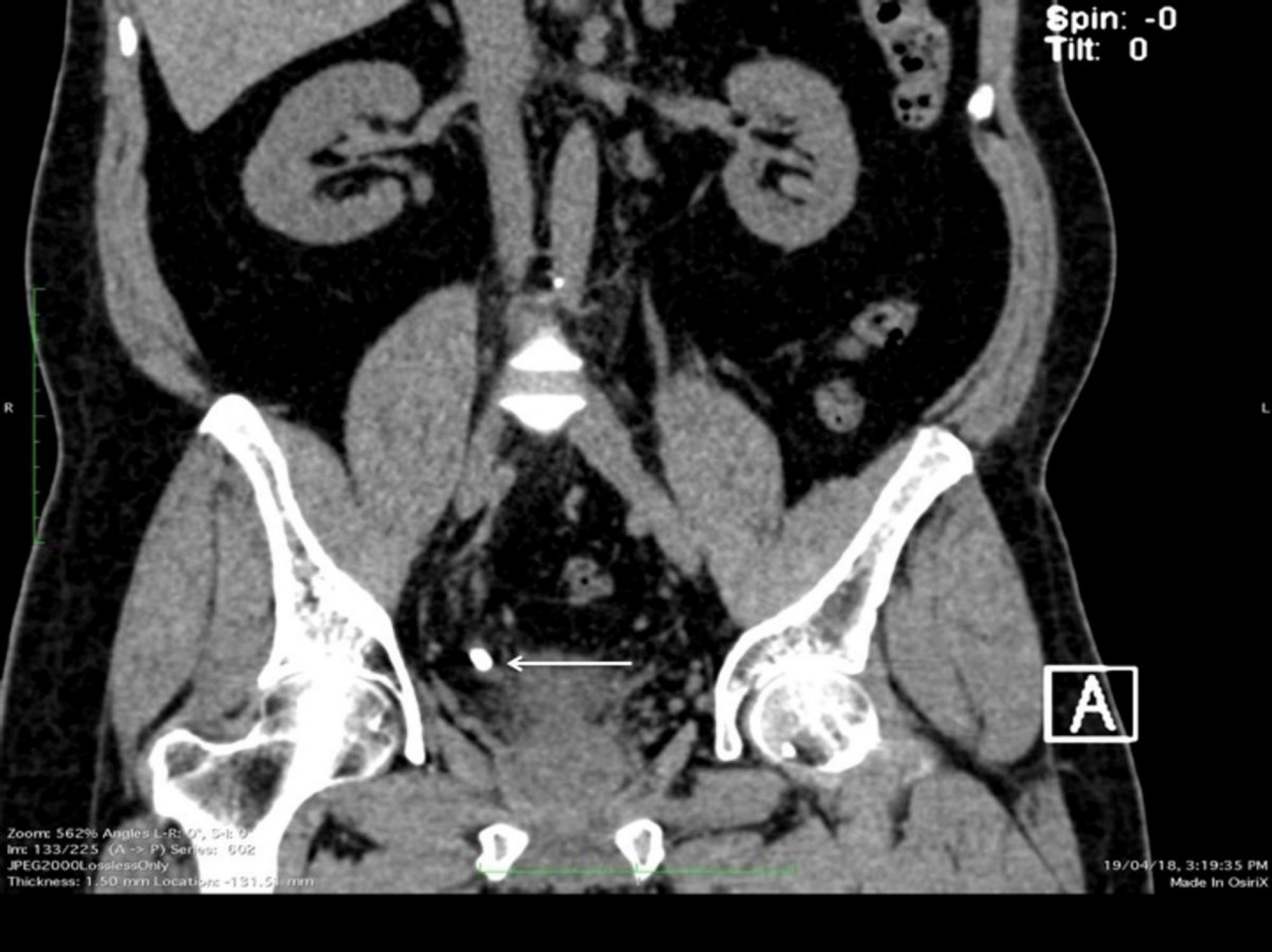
CT KUB (coronal view) - Revealed bladder calculi (arrow). CT: Computed tomography; KUB: Kidney ureter bladder.
